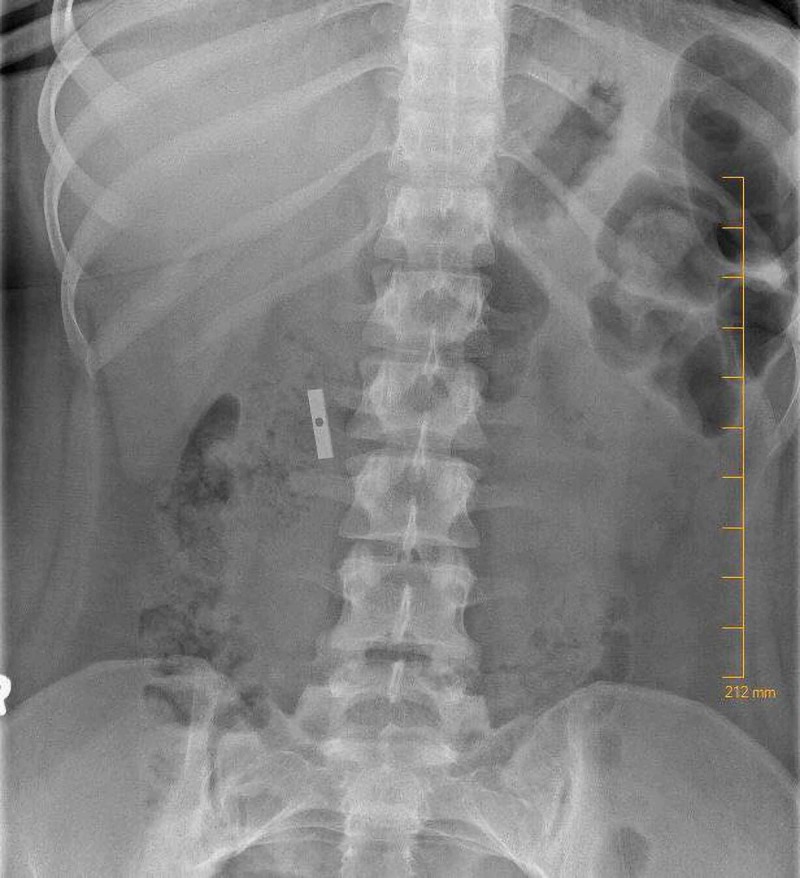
Anteroposterior radiograph demonstrates an isolated metallic object in the right upper quadrant consistent with suspected razor blade ingestion.
